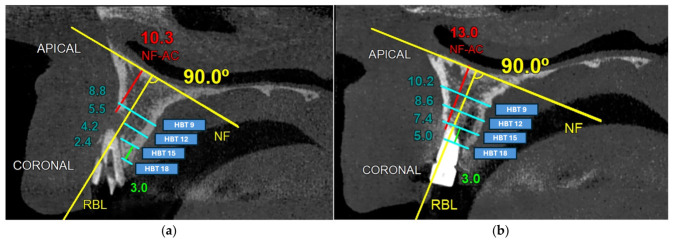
CBCT-based morphometric analysis at baseline (T0) and 12-month follow-up (T1). Horizontal bone thickness (HBT) was measured in the bucco-palatal direction at four apico-coronal levels 9, 12, 15, and 18 mm along the reference baseline (RBL). The nasal floor (NF) was used as a stable anatomical reference, and the nasal floor–alveolar crest distance (NF–AC) was assessed along the RBL. (a) Baseline tooth/socket site; (b) 12-month implant site.
